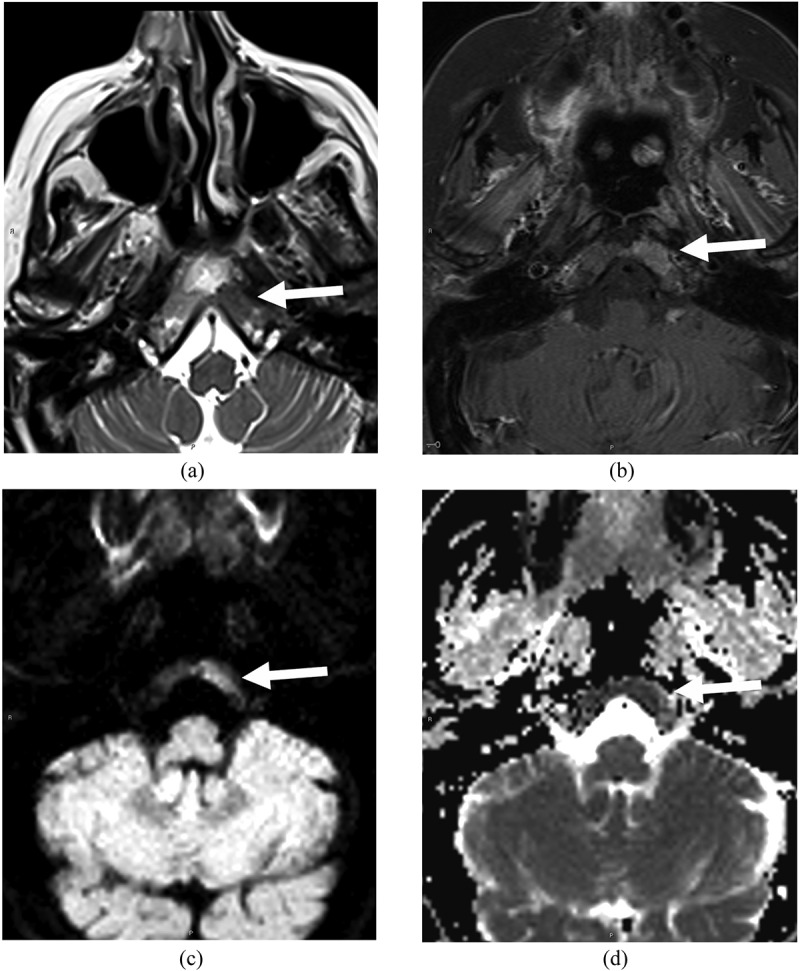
Axial MRI sinus showed disease infiltration in clivus (arrows) involving left Dorello’s canal with low MRI T2 signal (a) and high T1 signal with contrast enhancement (b) and restricted diffusion on DWI/ADC (c, d).
